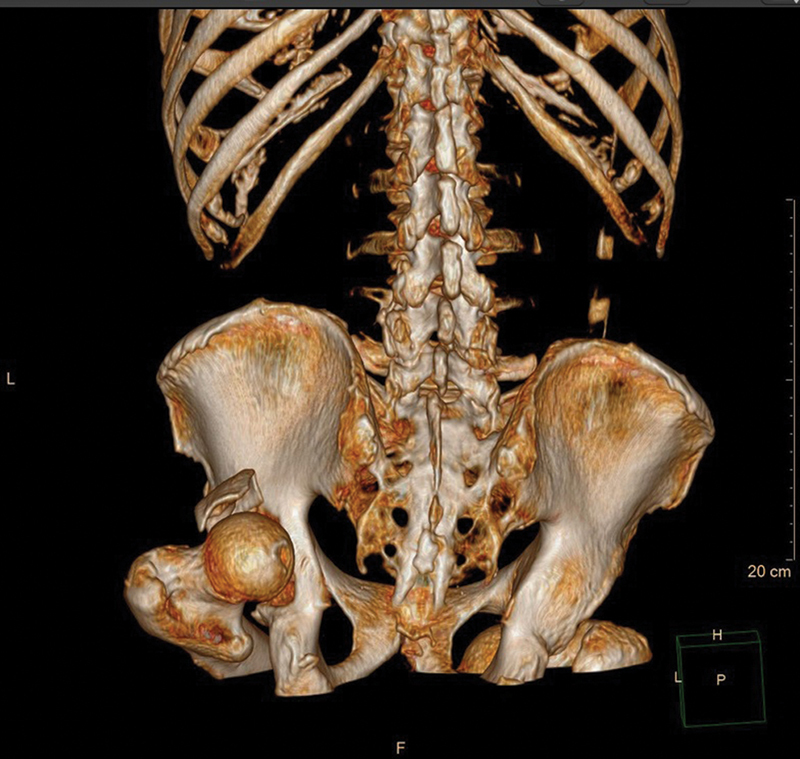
Computed tomography of the pelvis (3D reconstruction) showing the fracture of the posterior wall of the left acetabulum.
